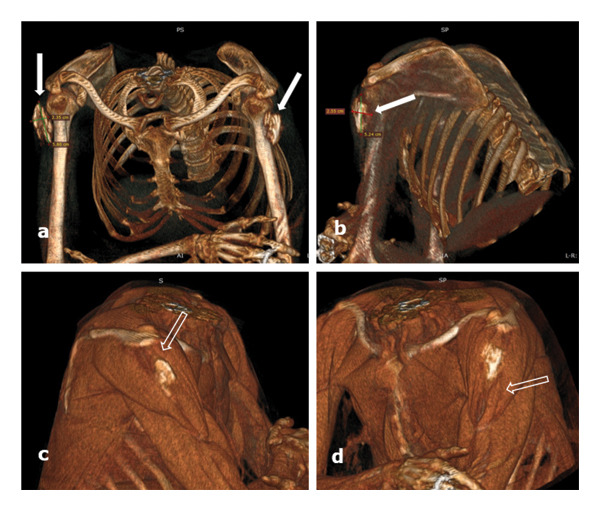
Chest MDCT (December 2023) native acquisition with 3D VR for bone (top images: (a) bilateral and (b) left shoulder) and small parts of the shoulders (bottom images: (c) right and (d) left): the global diameters of the ossified masses up to approximately 5.8 × 2.5 cm are better evaluated than by ultrasounds because of the irregular outer surface (white arrows); the ossified muscle fibers are interrupted and uneven, up to 5‐6 mm in length, suggesting the ossification of pathological fibers of the rhabdomyolysis type; the surrounding muscle structure is normal (empty arrows) and the fine cleavage space with the humeral periosteum is preserved.
